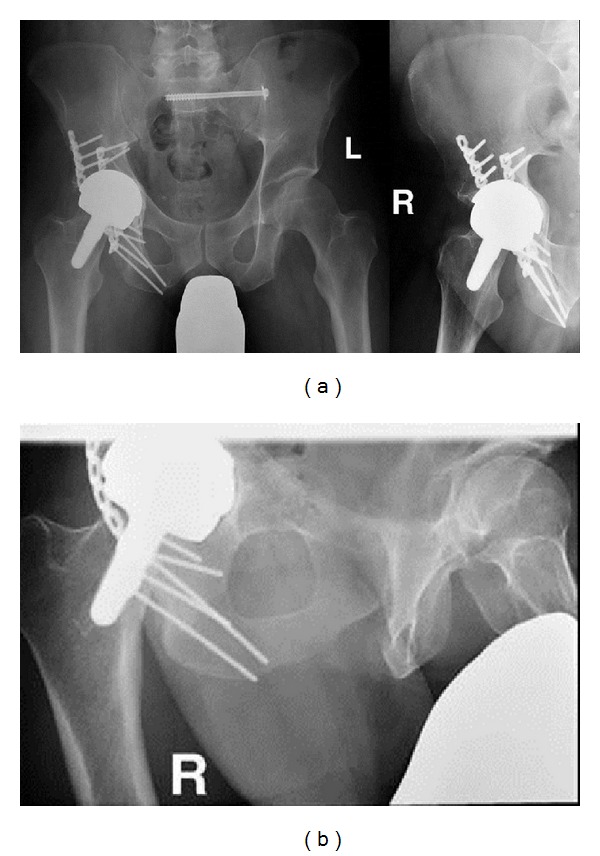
Postoperative X-ray 1 year after trauma showing bony healing in an anatomic position.
